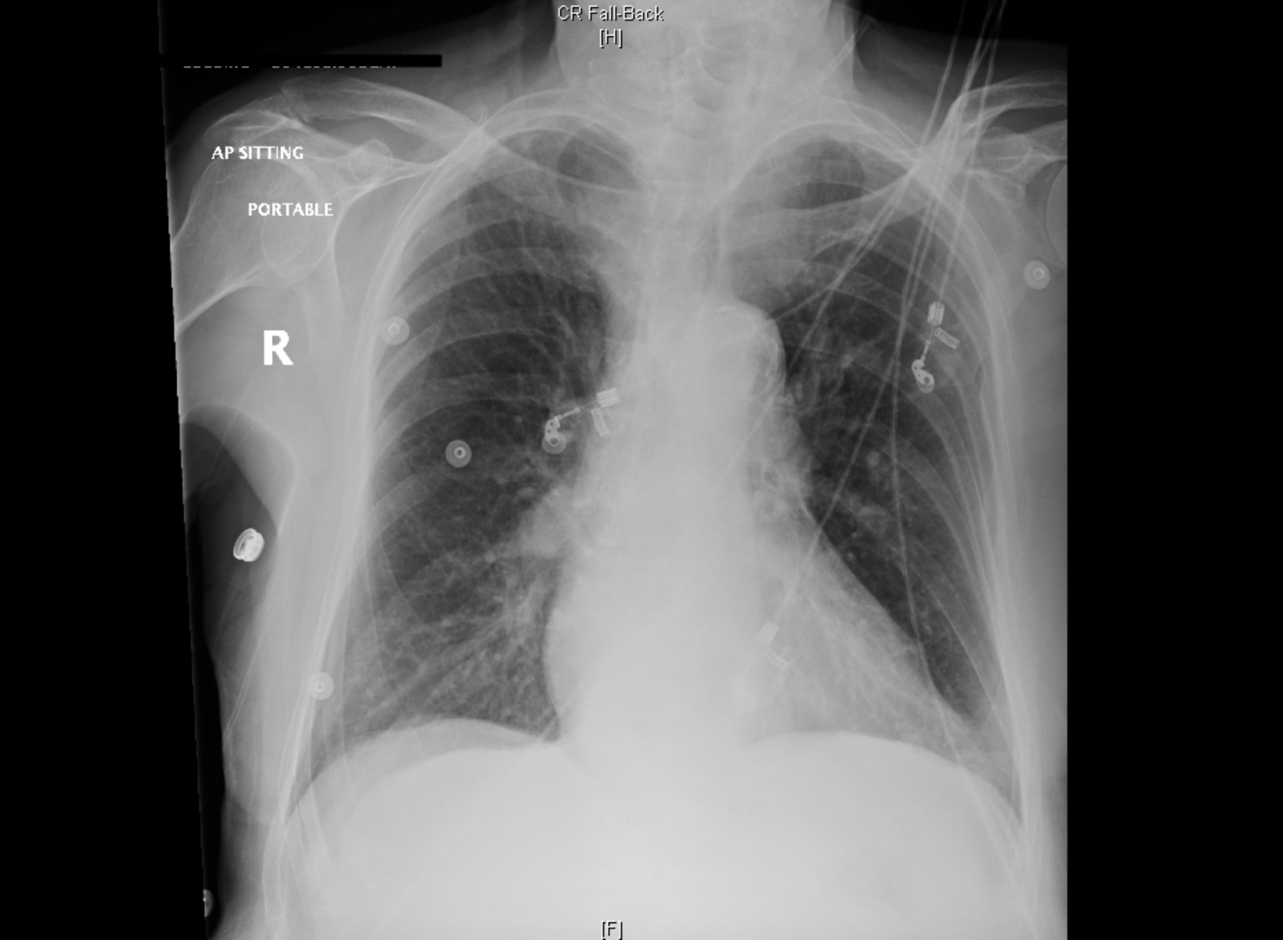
Portable chest X-ray (CXR).
